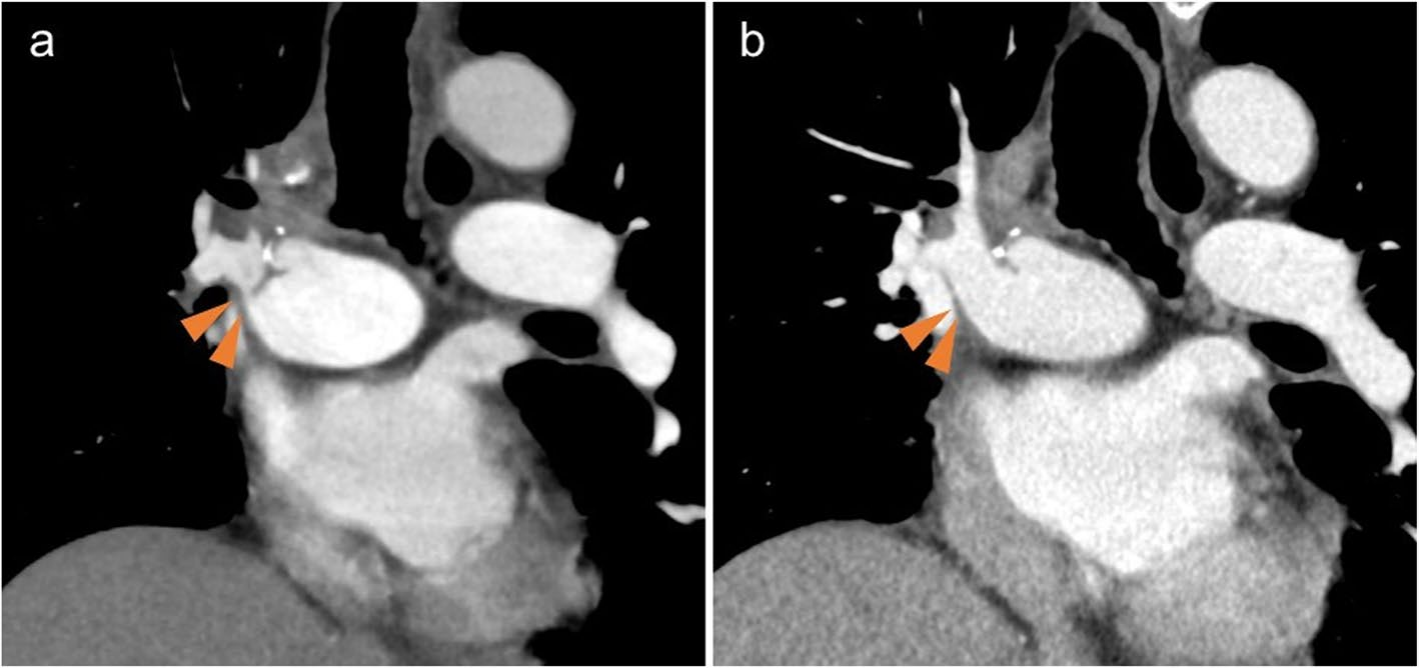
Contrast-enhanced CT after right lung transplantation and pulmonary artery plasty. Contrast-enhanced CT showed prominent stenosis at the anastomosis of the right pulmonary artery. Arrows indicate stenosis site (a). Contrast-enhanced CT after pulmonary artery plasty showed relief of the stenosis of the right pulmonary artery (b)
